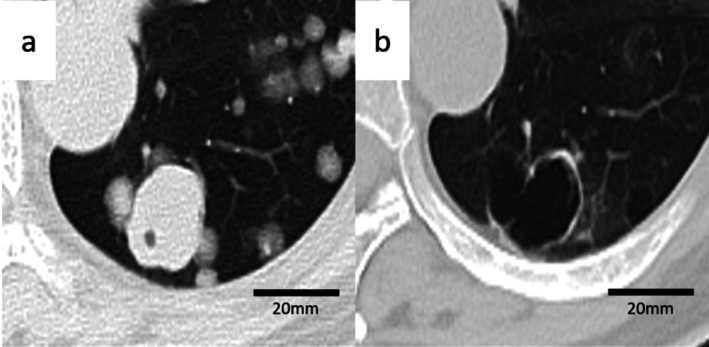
Magnified axial lung‐window CT after two EV cycles showing cavitation of a representative pulmonary metastasis with thin walls and near‐complete loss of solid components; scale bar = 20 mm. (a) Baseline before enfortumab vedotin (EV): Solid round metastatic nodule. (b) After two cycles of EV: The nodule has transformed into a thin‐walled cavity with near‐complete loss of solid components.
